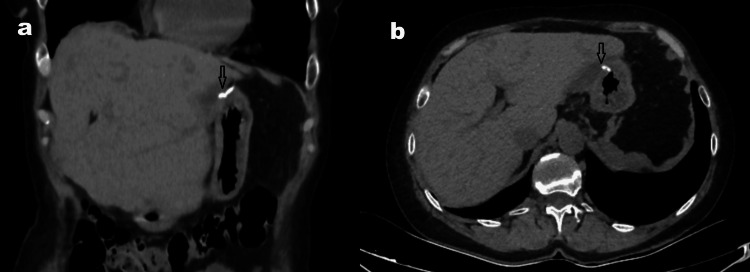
CT images obtained two years after surgery. CT images taken after a long follow-up period show decreased liver volume (a), the staple line in the anterior stomach (arrow), and an empty splenic lobe (a, b). No recurrence is observed.
